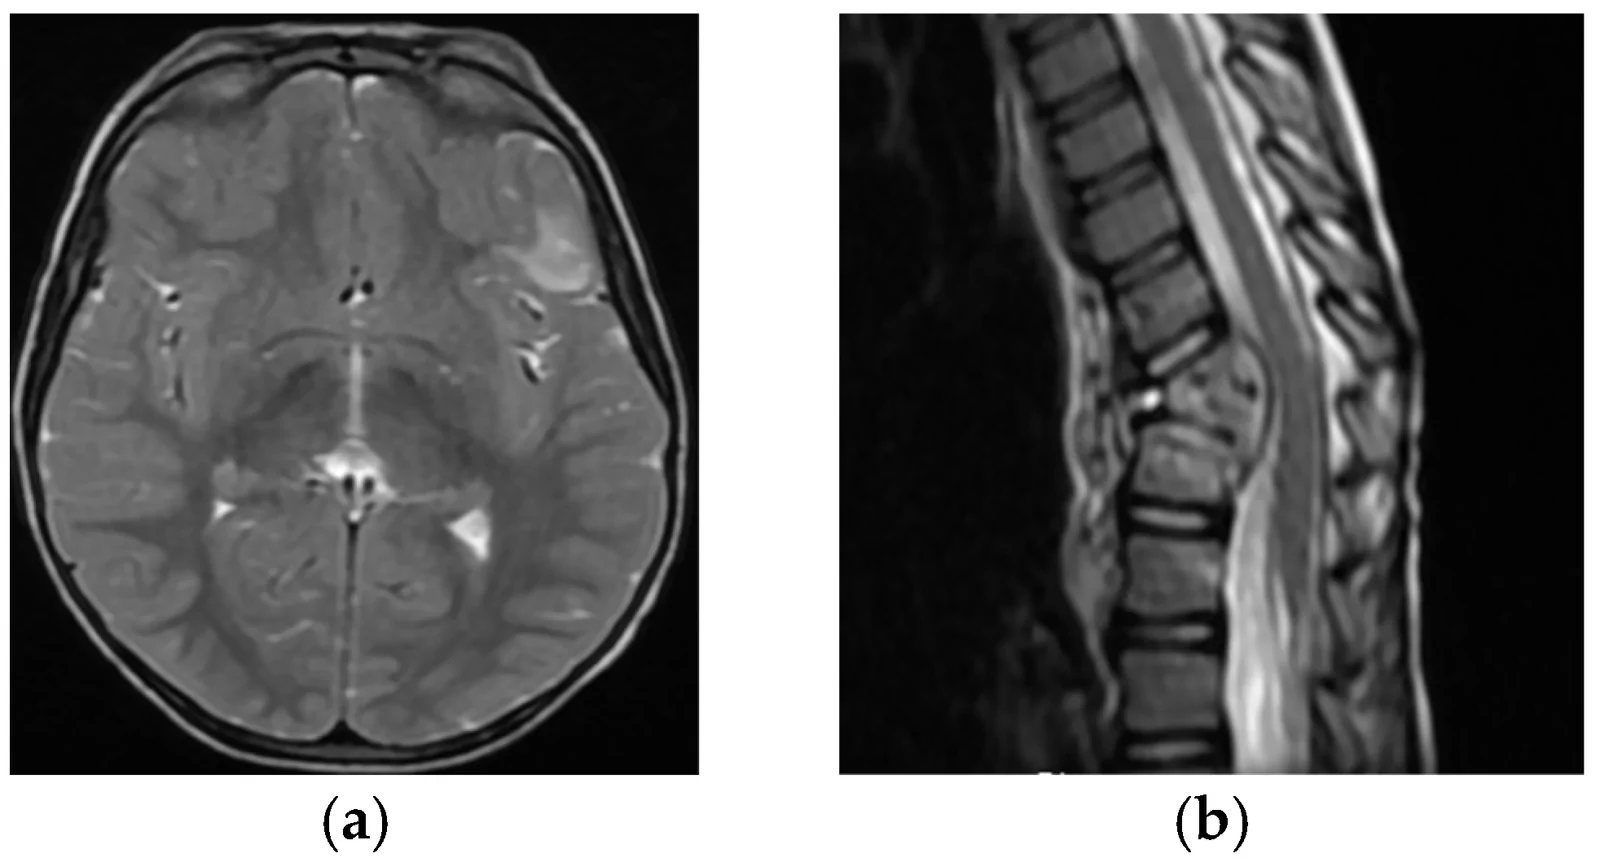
Three-month follow-up MRI of the brain and spine: (a) increased size of the left frontal tuberculoma; (b) enlargement of the paravertebral abscess, with involvement of the D11 vertebral body and initial involvement of D12, increased wedge-shaped deformity of D10, and progression of dorsal kyphosis.
